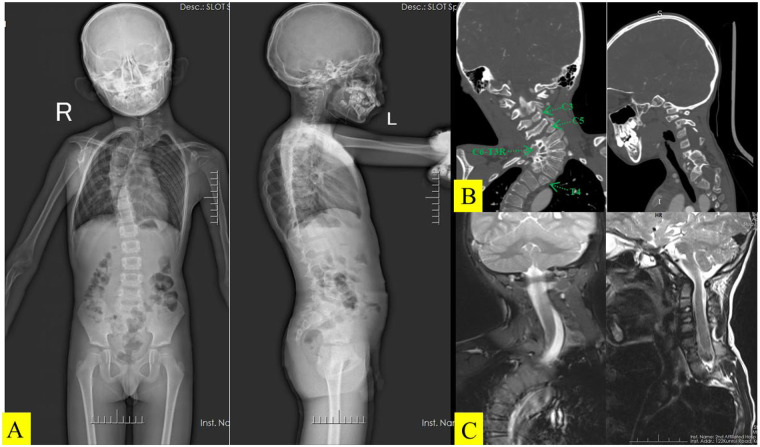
(A) Preoperative full-length anteroposterior and lateral spine X-ray images of the patient in the standing position. (B) Three-dimensional computed tomography imaging suggests that C3, C5, and T4 are butterfly vertebrae and that the area from C5 to T4 exhibits intervertebral fusion on the concave side. (C) Preoperative MRI of the cervical and thoracic spine suggests that there are no intraspinal malformations or degeneration of the spinal cord.
